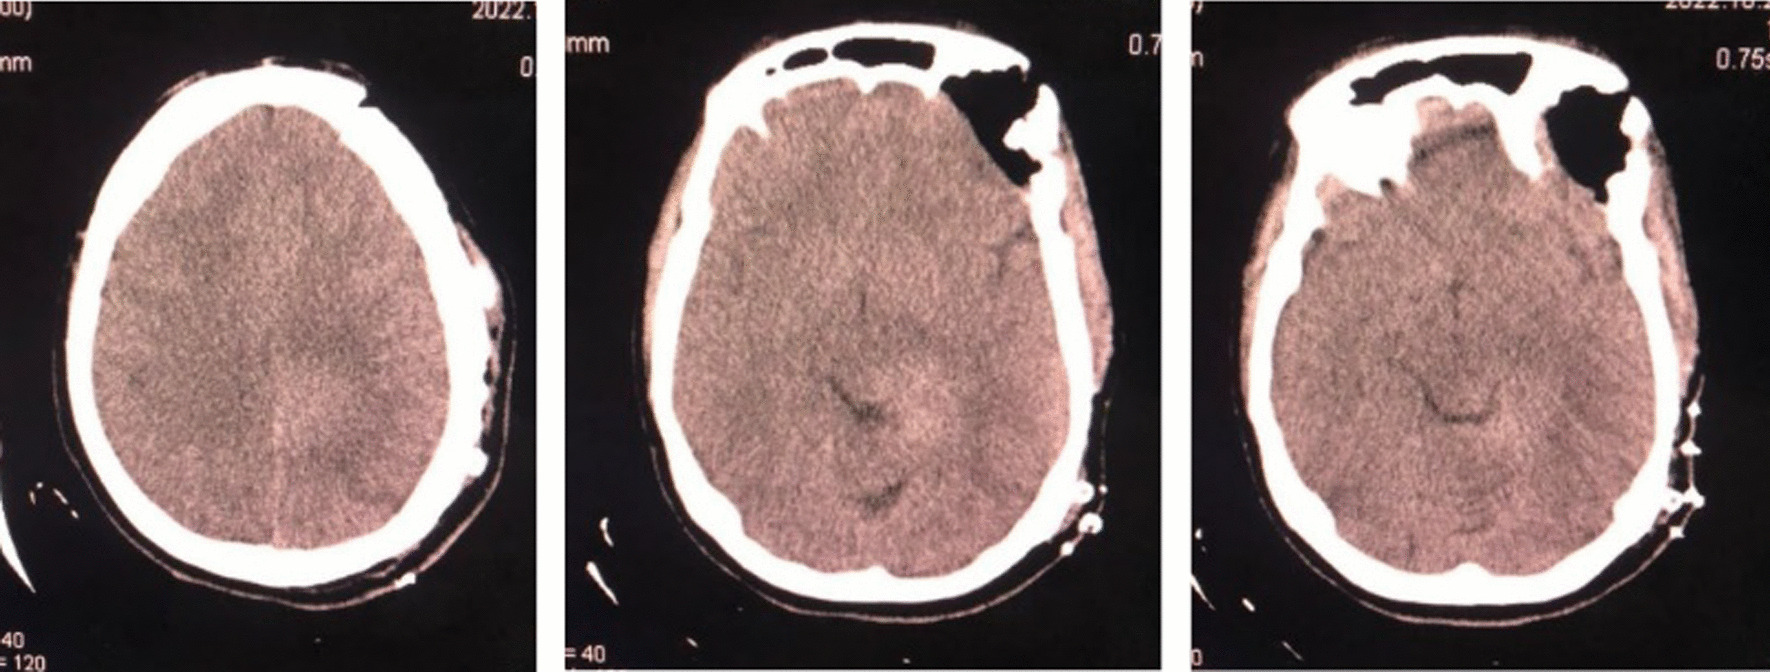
CT scan after surgery reveled normal findings with no residues
